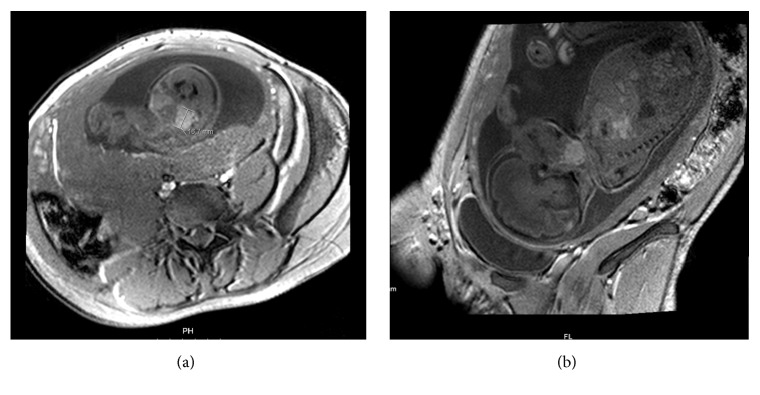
Fetal MRI showing fetal thyroid diffuse enlargement (a: coronal view; b: sagittal view).
